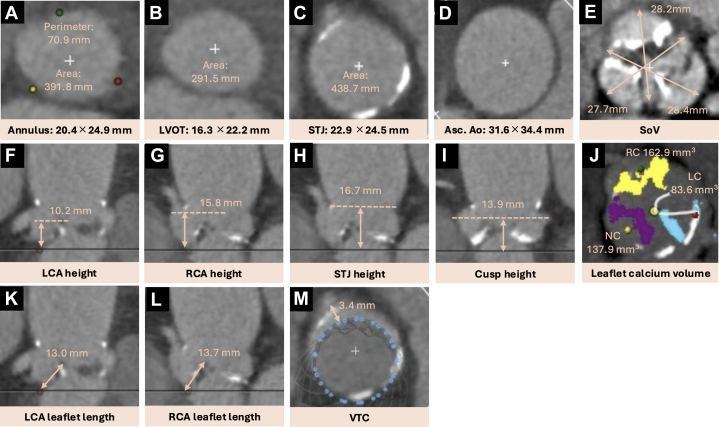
Preprocedural Computed Tomography Measurements (A to E) The anatomical features of the aortic valve were deemed suitable for implantation of a 26-mm Evolut FX valve. The overall risk of coronary artery obstruction was estimated to be low based on several parameters: (E) SoV diameters, (G) RCA height, (I) cusp height, and (J) calcium volume of the right coronary leaflet. However, (M) the VTC distance was measured at 3.4 mm, which was the only parameter below the established safety cutoff. Asc. Ao = ascending aorta; LC = left coronary; LCA = left coronary artery; LVOT = left ventricular outflow tract; NC = noncoronary; RC = right coronary; RCA = right coronary artery; SoV = sinus of Valsalva; STJ = sinotubular junction; VTC = valve-to-coronary.
